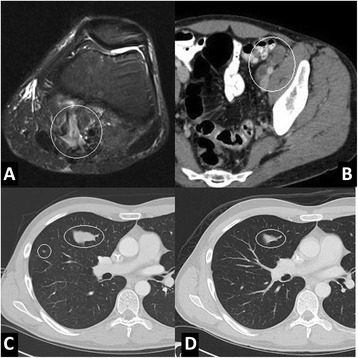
Radiologic response to cisplatin and paclitaxel. a Axial MRI demonstrates postoperative changes following surgical resection of popliteal mass without evidence of residual disease. b Axial contrast-enhanced CT images following neoadjuvant chemotherapy demonstrate decreased size of left external iliac nodes, consistent with response to therapy. On chemotherapy at (c) 1 month and (d) 4 months after presentation, both the right lung mass and nodule have markedly improved consistent with response to therapy
